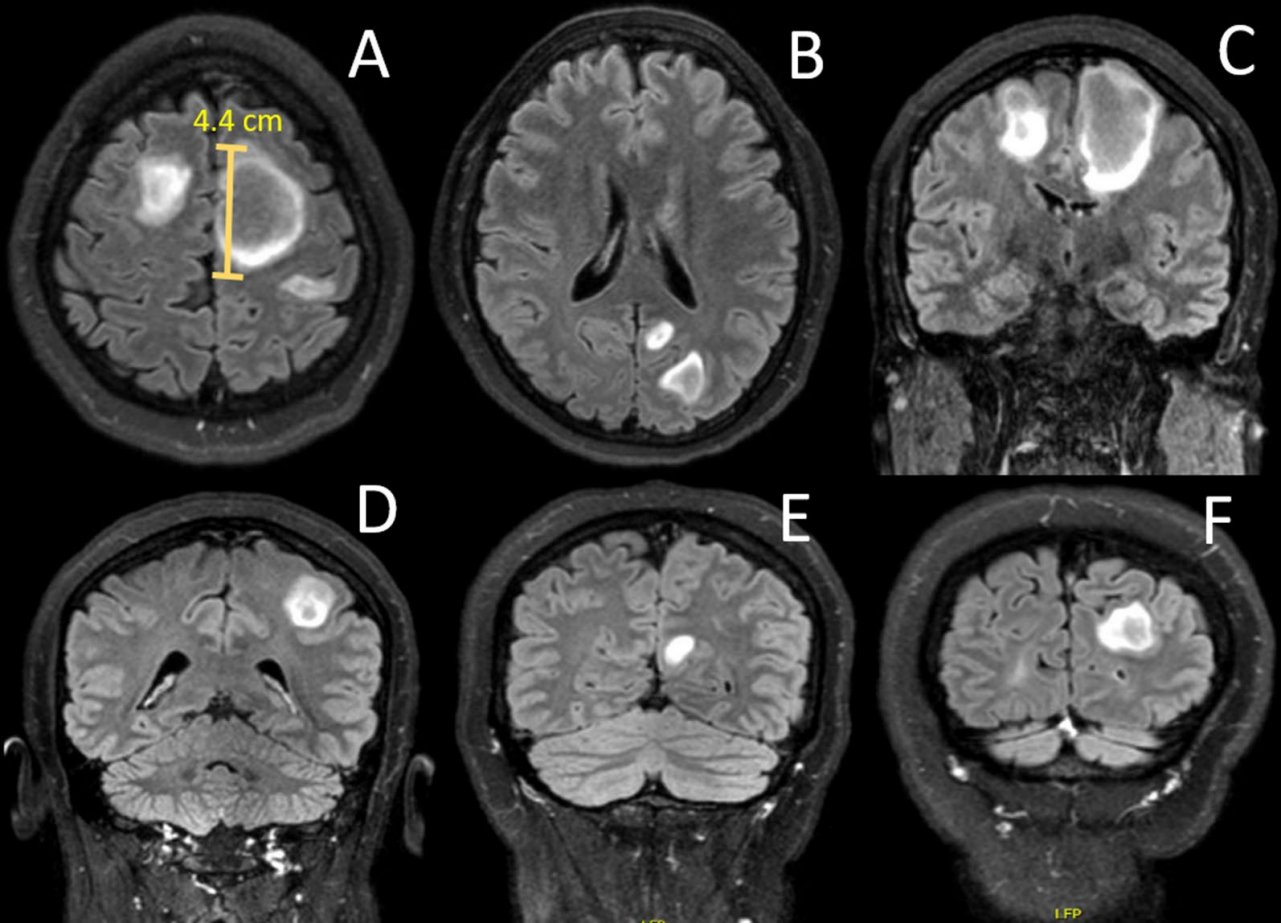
Axial T2 fluid-attenuated inversion recovery (T2 FLAIR) MRIs at admission showing five large juxtacortical lesions supratentorially. One lesion in the right cerebral hemisphere and four in the left cerebral hemisphere. The largest lesion was located in the left frontal lope and measured approximately 4.4 cm in diameter (A). A + B Axial sections. C–F Coronal sections
